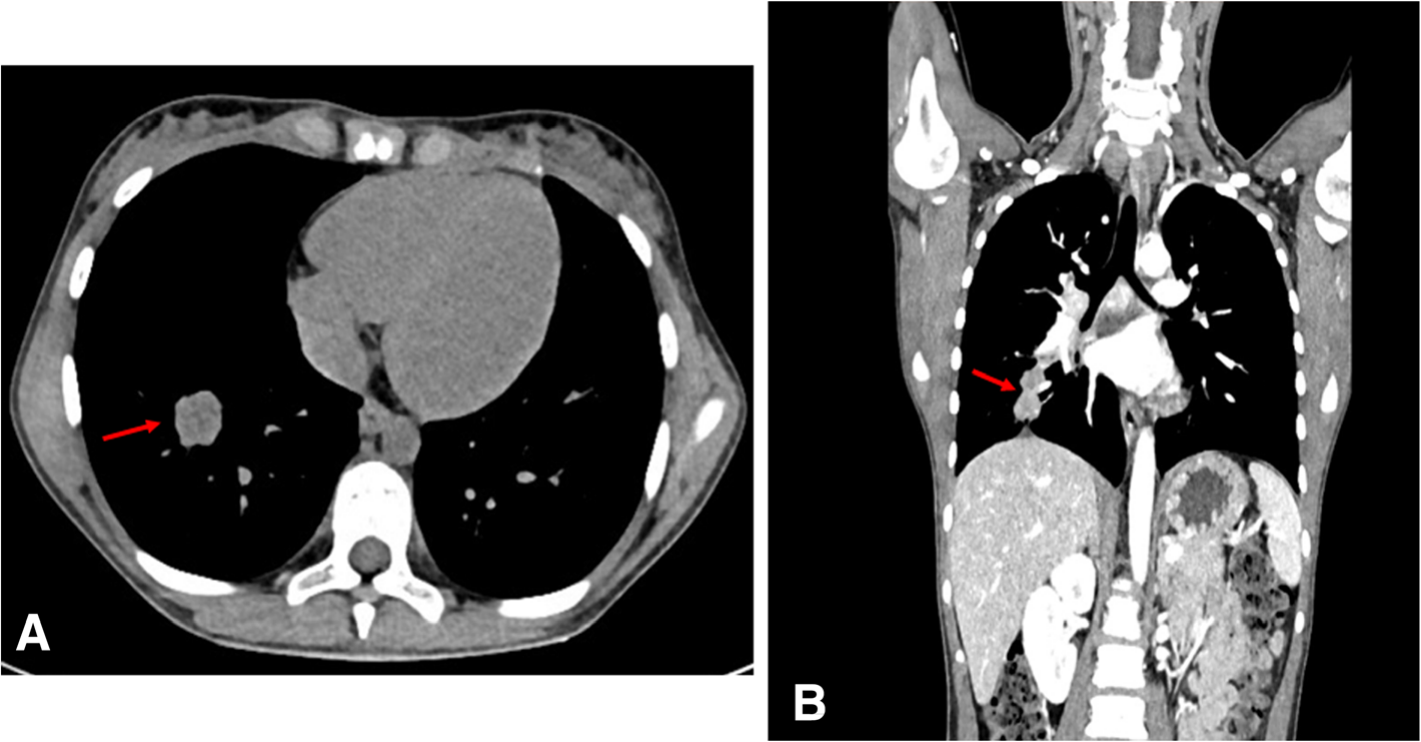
Lung adenocarcinoma in the lower lateral basal segment of the right lobe (red arrow) in our 10-year-old white girl on axial (a) and coronal (b) computed tomography scan
